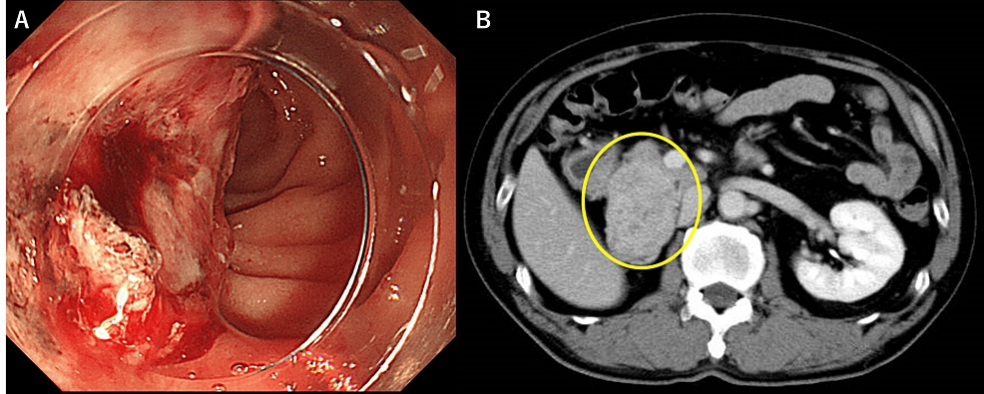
Endoscopic image of duodenum and contrast-enhanced abdominal computed tomography (A) Endoscopic image of duodenum. Invasion from a tumor of the pancreatic head, (B) Computed tomography showing hypervascular tumor of pancreas head.
